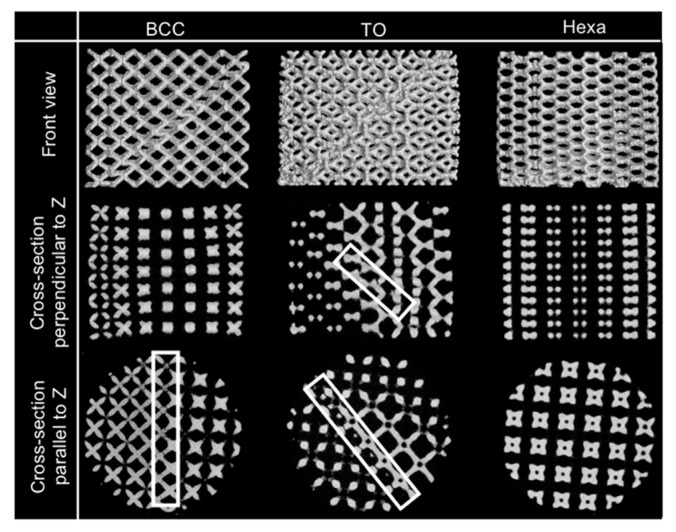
X-ray computed tomography (CT) reconstruction models and cross-section images of 20% compressed lattice structures.
